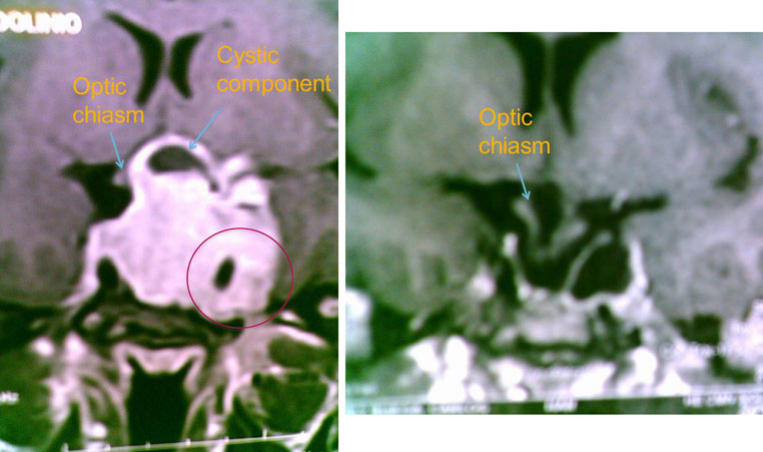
Gadolinium-enhanced, T1-weighted MRI scans (coronal view) of pituitary tumor at diagnosis (*left*) and after 6 months of treatment with an SSA and dopamine agonist
